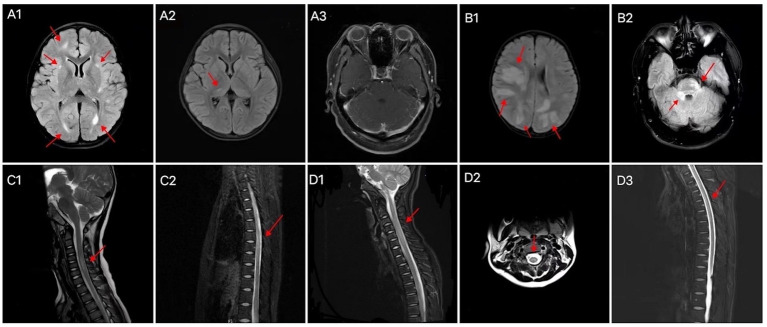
Brain MRI characteristics of patients with (A) GFAP-IgG-and (B) MOG-IgG-associated myelitis. Abnormal signals in the right frontal lobe, bilateral lateral posterior horn of the ventricle, and bilateral basal ganglia areas (A1); abnormal signals in the right thalamus (A2); bilateral cerebellar multiple strip enhancement (A3); multiple abnormal signals in the cortex/subcortex (B1); abnormal signal in the right pontocerebellar commissure and pons (B2). Spinal cord MRI characteristics of patients with GFAP-IgG-associated myelitis (C1, C2) and MOG-IgG-associated myelitis (D1–D3). LETM of the cervical cord (C1), LETM of the thoracic cord (C2), LETM of the cervical cord (D1), “H sign” (D2), and LETM of the thoracic cord (D3). LETM, longitudinally extensive transverse myelitis; MRI, magnetic resonance imaging; GFAP, glial fibrillary acidic protein; IgG, immunoglobulin G; MOG, myelin oligodendrocyte glycoprotein.
